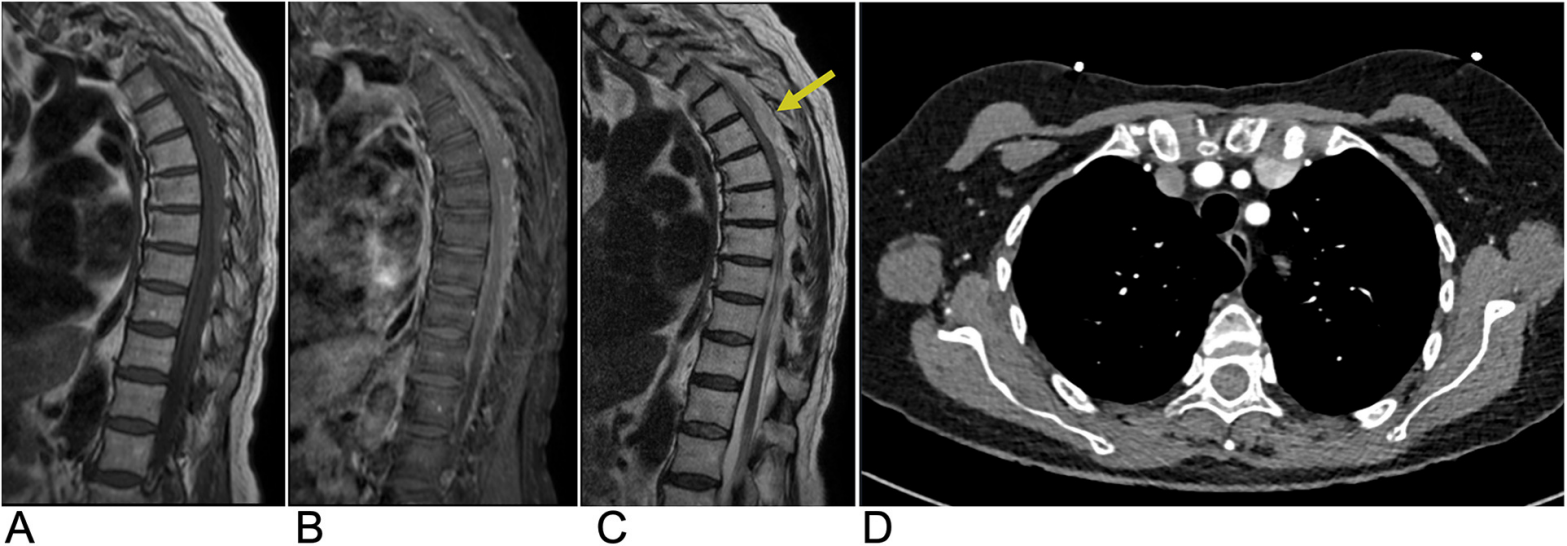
Epidural hematoma. Sagittal T1 (A), sagittal T1 + C (B), and sagittal T2 (C) MRI as well as an axial CT (D) of the thoracic spine demonstrating a T1 isointense, T2 hyperintense (yellow arrow), and CT isodense collection in the dorsal epidural space of the midthoracic spine representing epidural hematoma with associated mass effect on the spinal cord.
